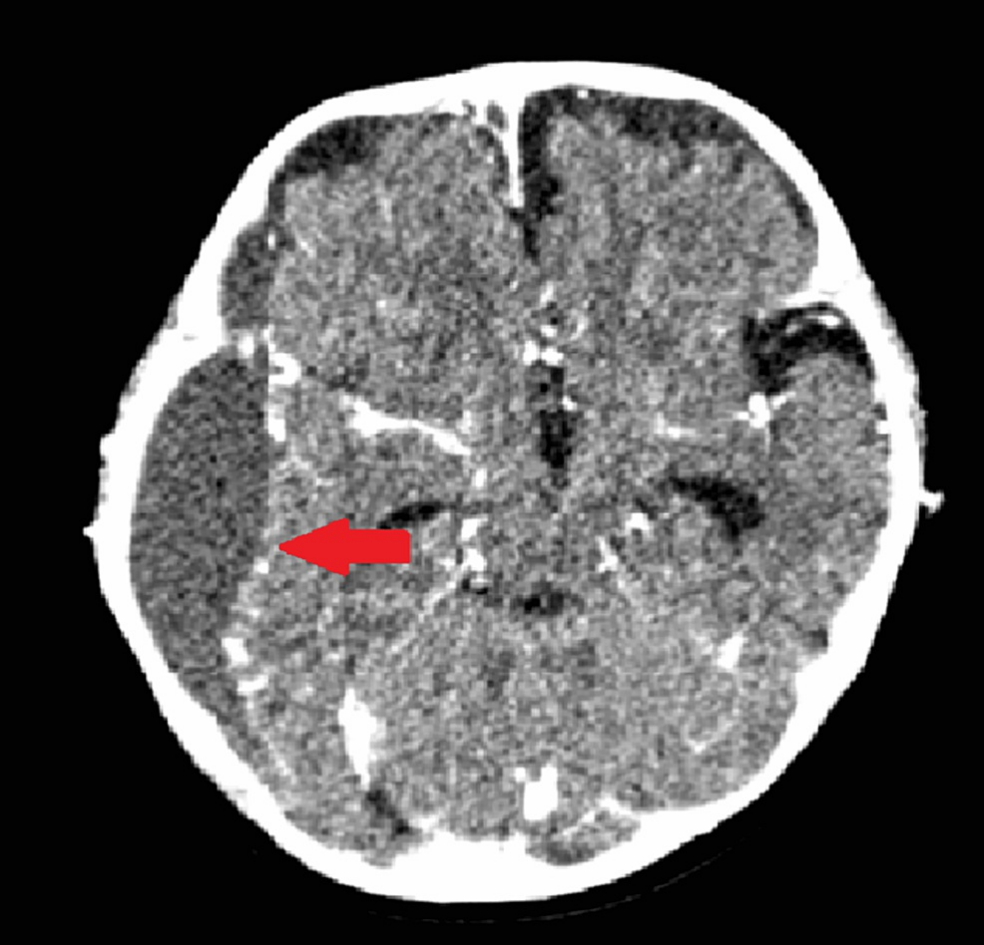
CECT brain axial view (parenchymal window) showing large right frontotemporal extradural empyema (measuring 8.9 × 2.4 × 9.2 cm) causing mass effect and midline shift. CECT: contrast-enhanced computed tomography
